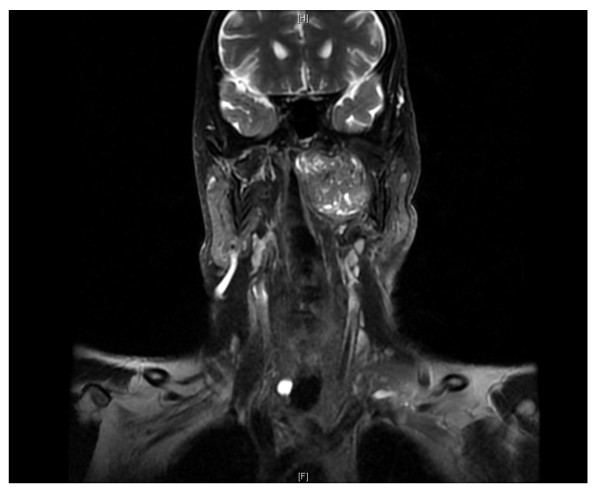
MRI showing 5 × 6 × 3.3 cm enhancing left parapharyngeal mass compressing the upper internal jugular vein.
